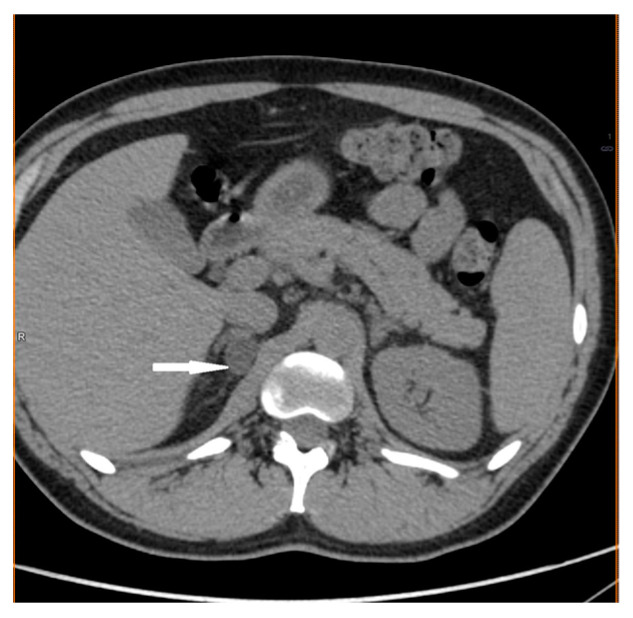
Adrenal cortical adenoma. Abdomen CT, transverse cross-section.
